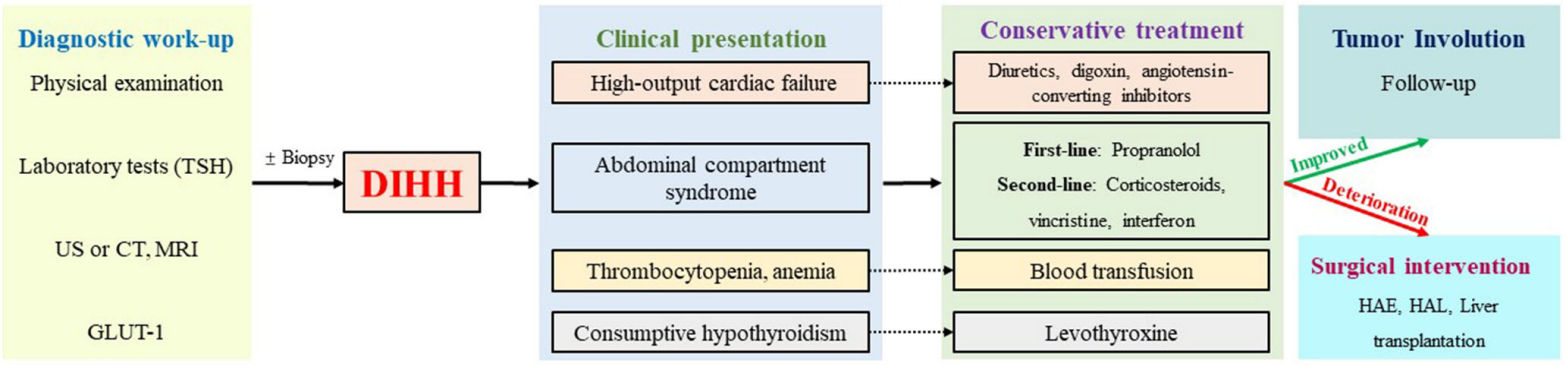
The diagnosis and treatment algorithms for DIHH. US, ultrasonography; CT, computed tomography MRI, magnetic resonance imaging; TSH, thyroid stimulating hormone; GLUT-1, glucose transporter isoform 1; HAE, Hepatic artery embolization; HAL, Hepatic artery ligation.
